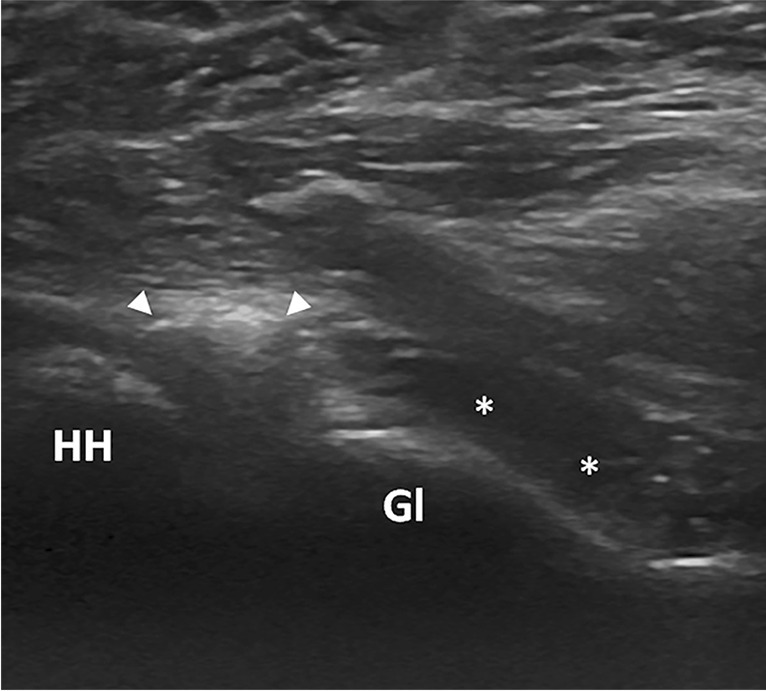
Glenohumeral joint hydrodistension with capsular rupture in a 58-year-old female. Sonographic image shows a distended posterior joint recess (arrowheads), with extraarticular fluid (*) extending medially following capsular rupture. HH, humeral head; Gl, glenoid
